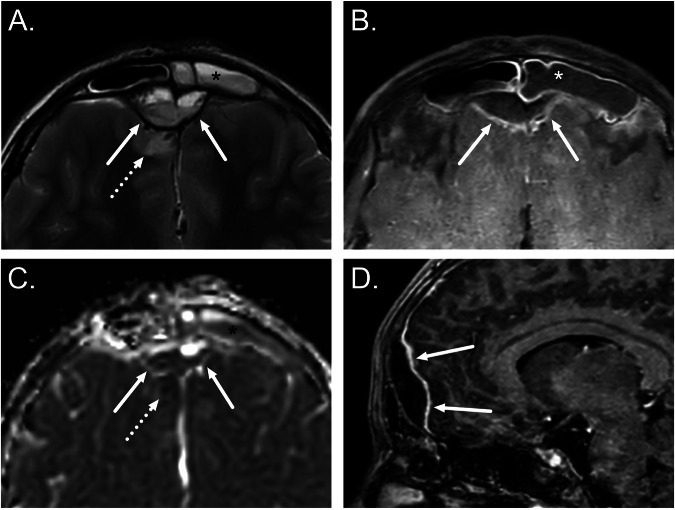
Frontal sinusitis complicated by an epidural abscess, pachymeningitis, and cerebritis. Axial T2-weighted (A), axial fat-suppressed post-contrast T1-weighted (B), and sagittal post-contrast T1-weighted (D) images and an axial ADC map (C) demonstrate purulent fluid in the left frontal sinus (asterisks) and an epidural purulent collection with dural enhancement (arrows). Edema (A) and restricted diffusion (C) in the adjacent brain tissue (dotted arrows) suggest cerebritis
